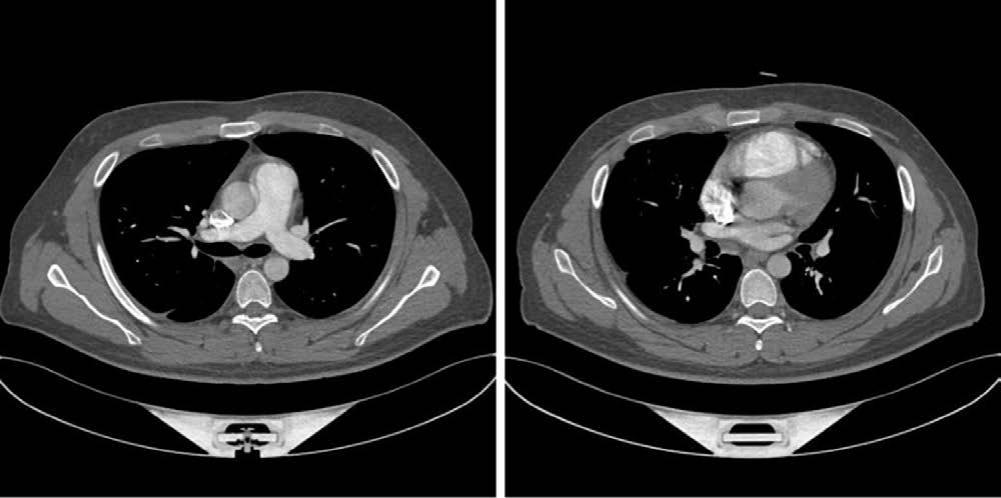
CTA of the pulmonary artery. CTA = computed tomography angiography.
